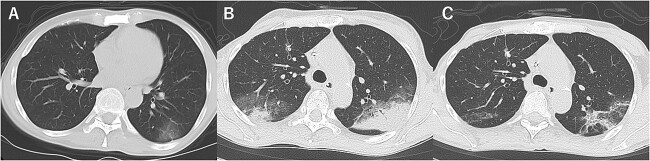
A series of chest computed tomography images. (A) On admission—ground-glass opacity in the left lower lung lobe. (B) Day 20—Sub-pleural, multiple consolidations in both upper lungs. (C) Day 34—fading consolidation.
